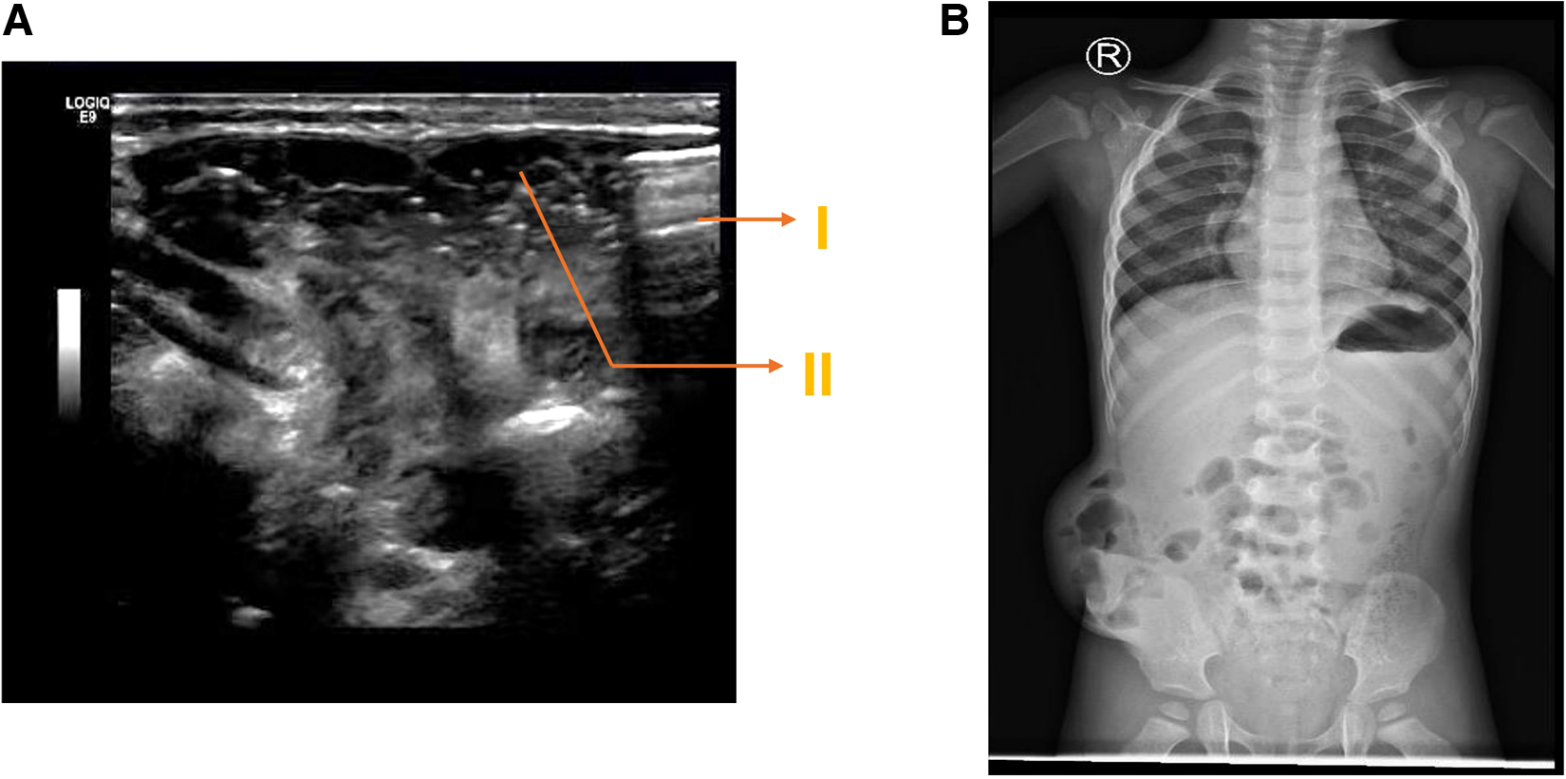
(A) Abdominal ultrasound prompts TAWH before operation. (I: muscle and peritoneal layer rupture caused by trauma; II: intestinal contents in the abdominal cavity protrude subcutaneously through muscle and peritoneal rupture). (B) Abdominal x-ray film before surgery.
